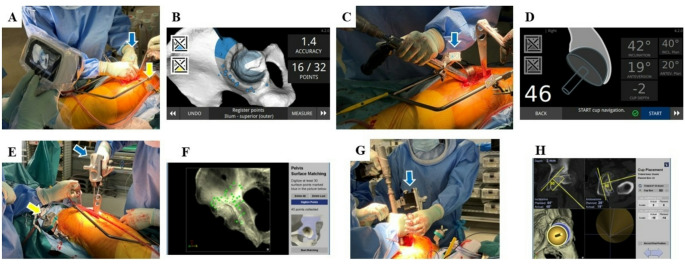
Portable CT-based navigation system and CT-based navigation system. A Portable CT-based navigation system. Intraoperative image during registration. The pelvic surface is palpated with a pointer with a blue tag (Indicated by the blue arrow). A yellow tag is attached to the pelvis (Indicated by the yellow arrow). B Navigation screen during surface matching. C The blue tag is attached to the reamer (Indicated by the blue arrow). D Navigation screen during reaming and cup placement. The angle and position of the cup was checked. E CT-based navigation system. Intraoperative image during registration. The pelvic surface is palpated with a pointer (Indicated by the blue arrow). The tracker is attached to the pelvis (Indicated by the yellow arrow). F Navigation screen during surface matching. G Navigation attached to the reamer (Indicated by the blue arrow). H Navigation screen during reaming and cup placement. The angle and position of the cup was checked
